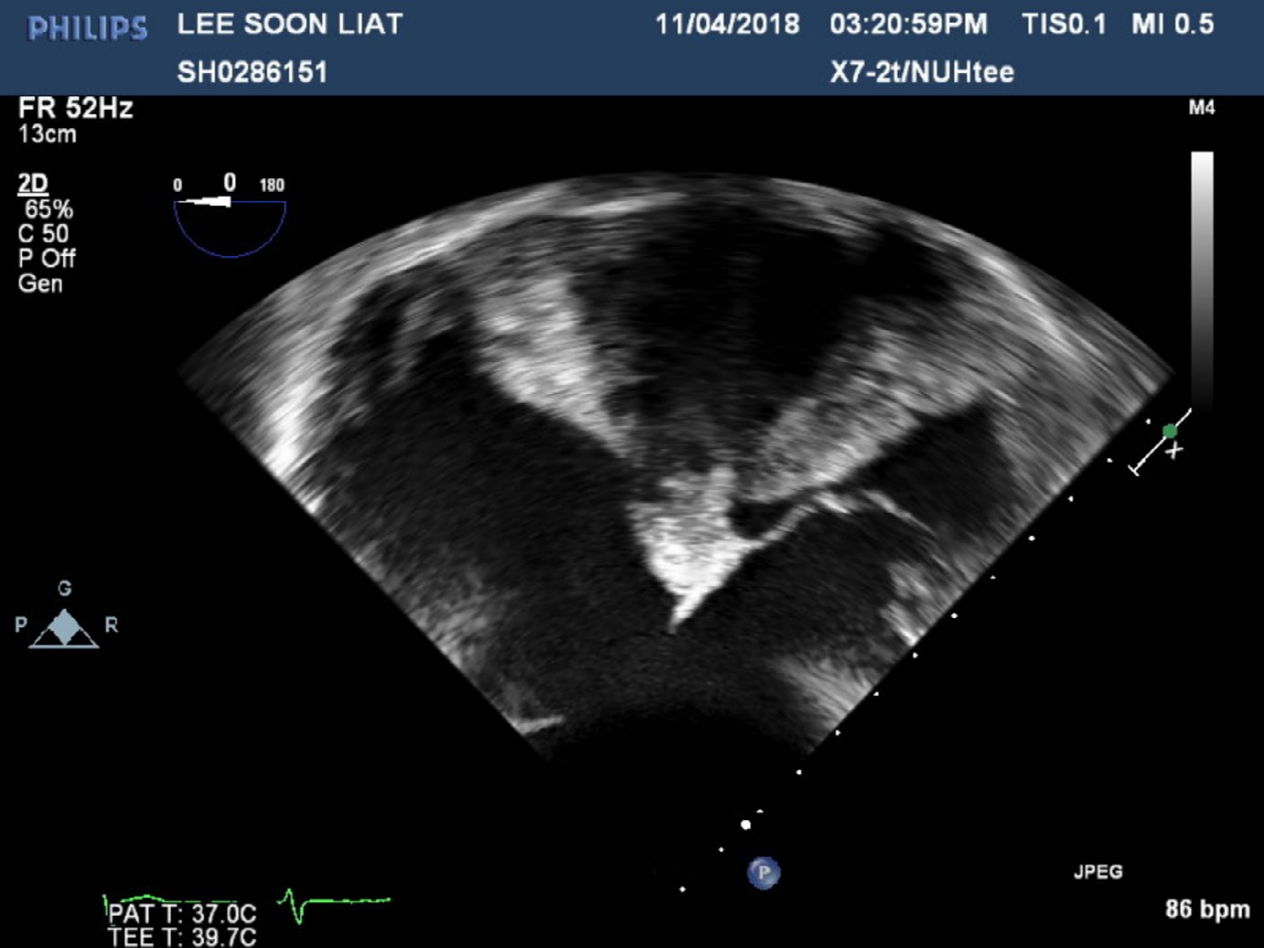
Preoperative Transesophageal Echocardiogram showing ASD
